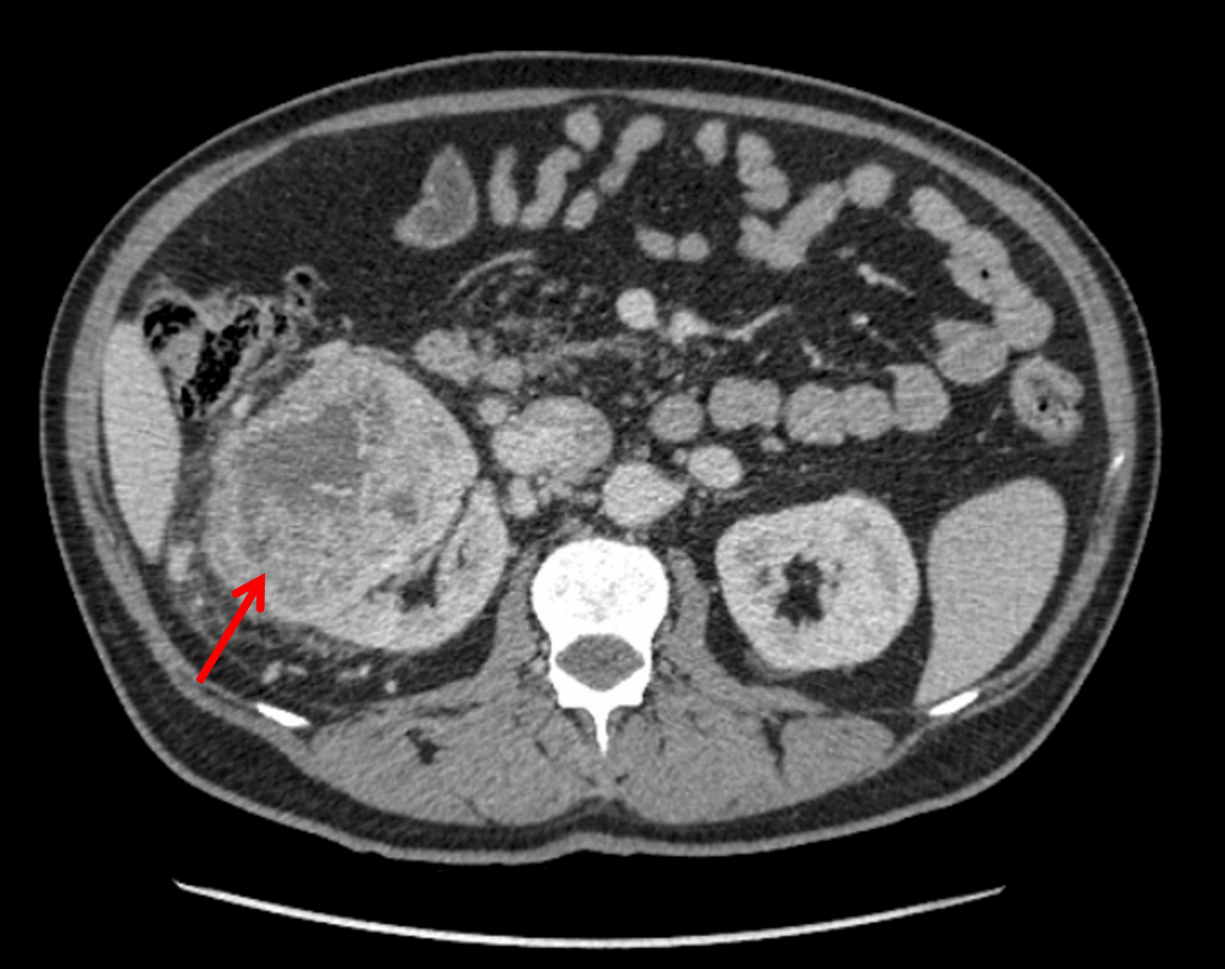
Abdominal CT (at initial diagnosis) Portal venous phase, axial view. CT showing a large heterogeneous right renal mass (135×115 mm). CT: computed tomography
